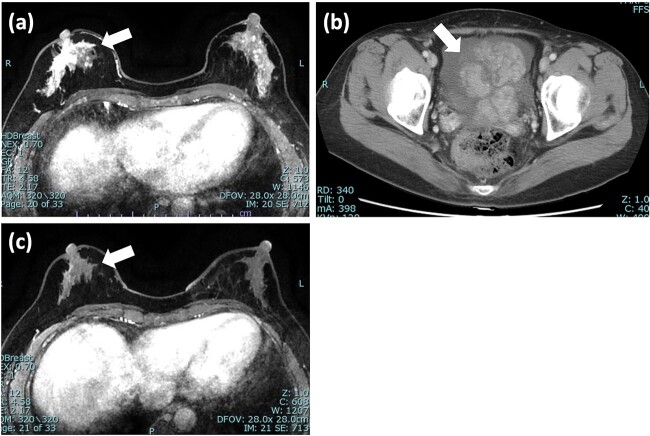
Imaging tests of right breast cancer and ovarian tumor. (a) MRI of the breast showed heterogeneous enhanced lesion below the nipple; (b) CT scan of pelvis revealed an ovarian tumor with increased ascites; (c) MRI after 5 months showed significant reduction in the size of tumor and diminished enhancement. MRI: magnetic resonance imaging; CT: computed tomography.
